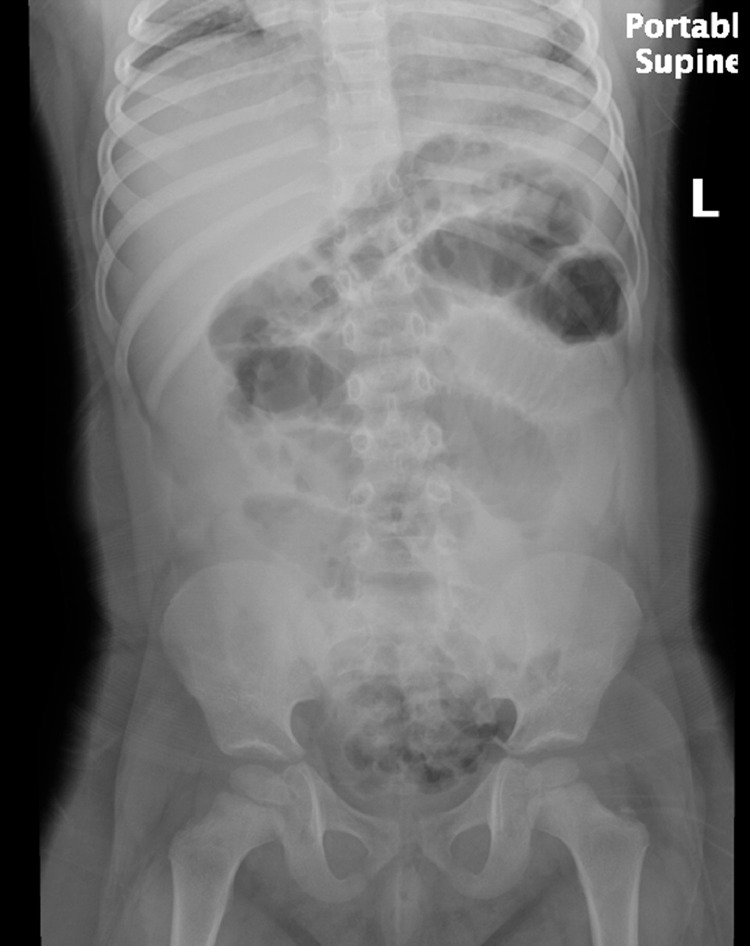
Supine abdominal radiograph demonstrates abnormal gaseous distention of small bowel loops throughout the abdomen, compatible with small bowel obstruction. No pneumoperitoneum or pneumatosis intestinalis.
